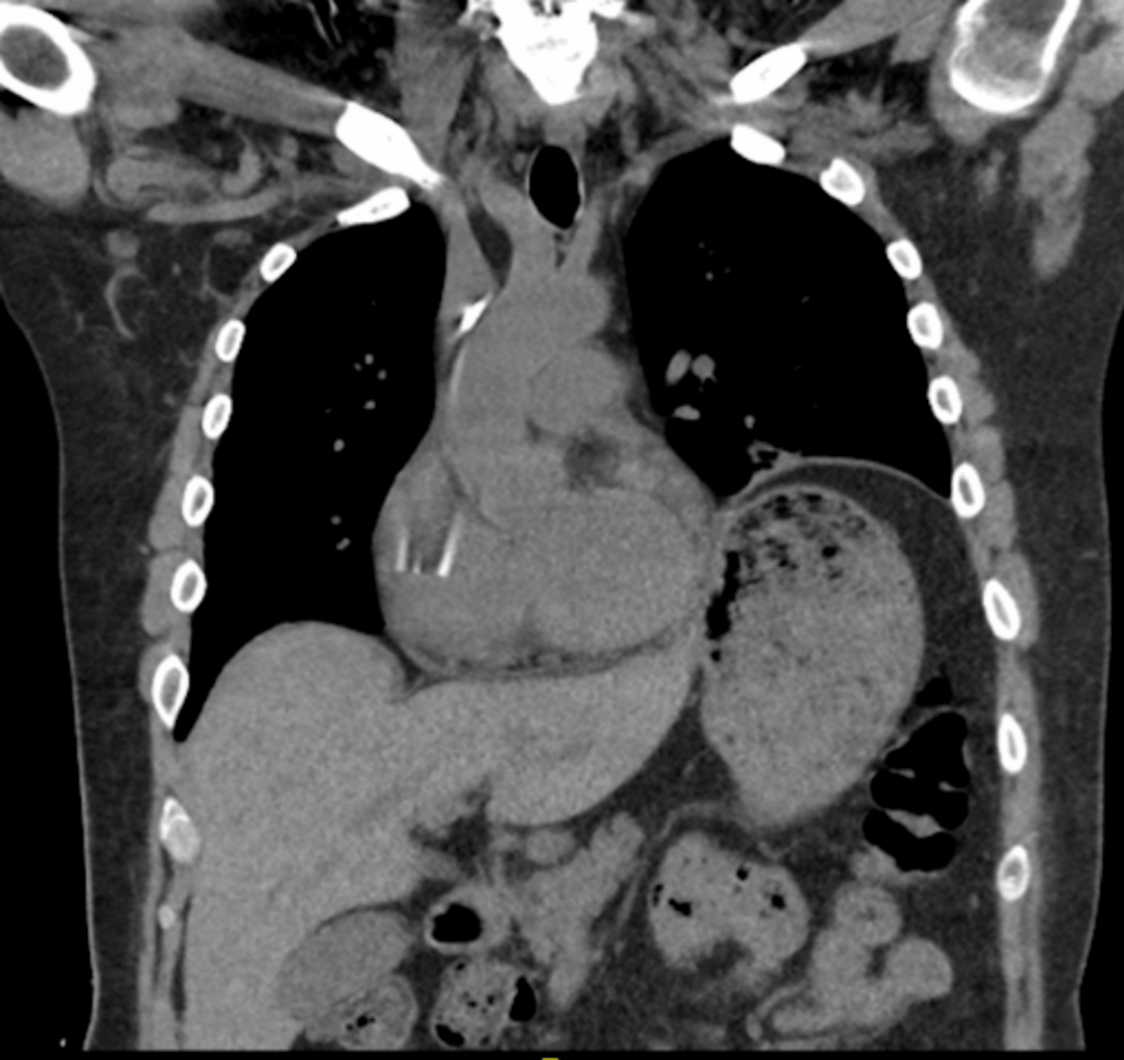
Computed tomography of the thorax reveals asymmetric elevation of the left hemidiaphragm and left basilar atelectasis or scarring.
